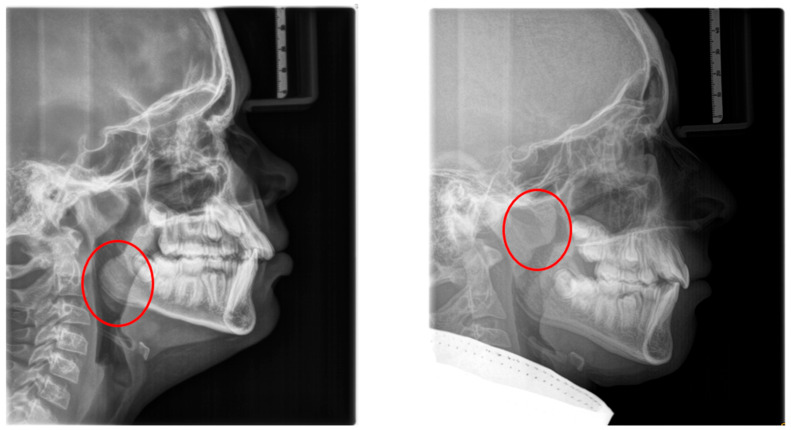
Radiographic lateral cephalograms: (left) red circle: enlarged tonsils; (right) red circle: enlarged adenoids.
